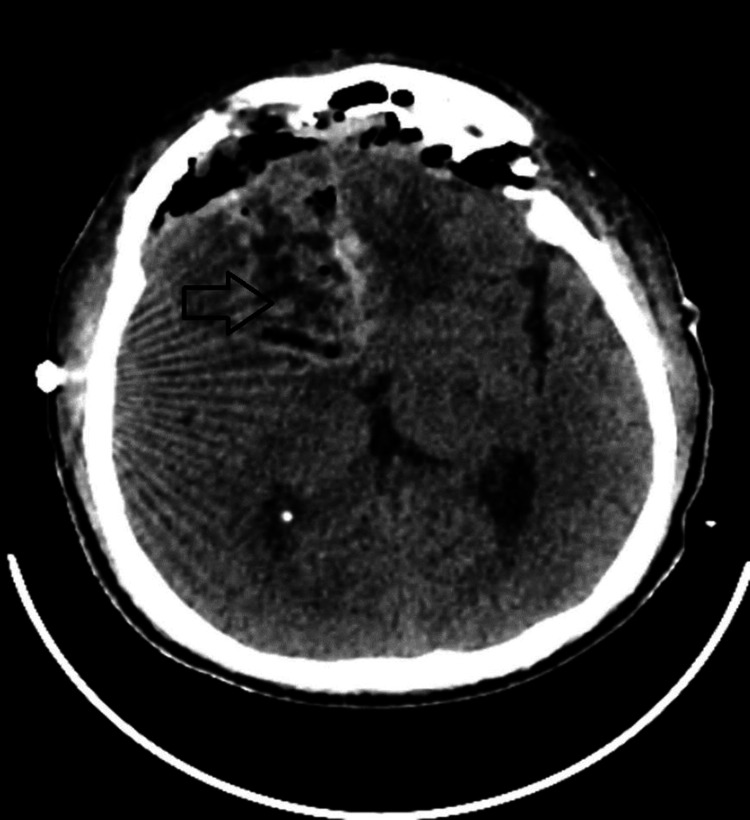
Depicting post-operative CT brain (plain); complete excision of the abscess wall and no operative site hematoma.
